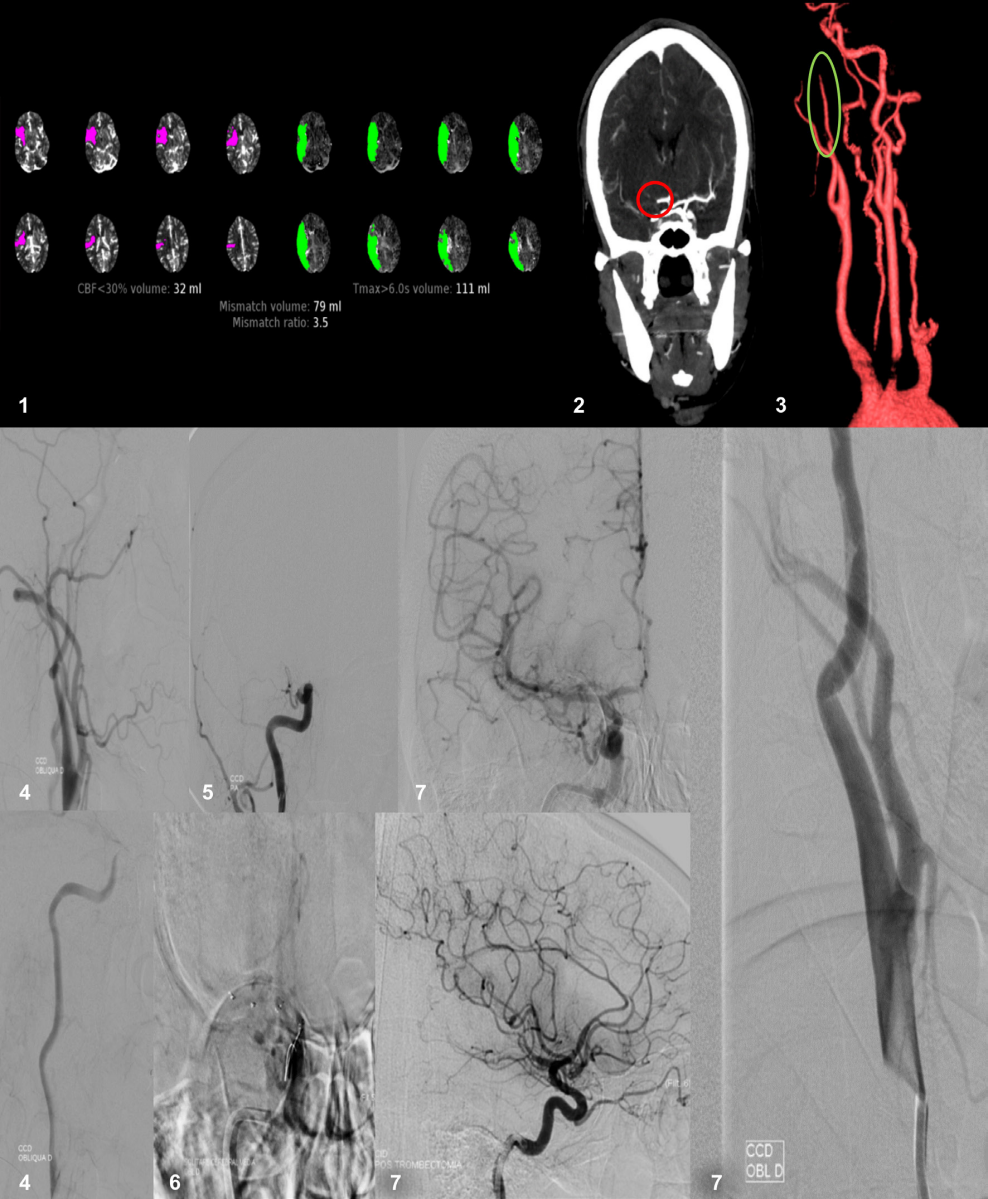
Patient that was admitted with acute stroke due to tandem occlusion and underwent mechanical thrombectomy. 1. Perfusion CT showing an acute stroke with large penumbra. 2. Median cerebral artery occlusion on CTA. 3. Internal carotid dissection with “pencil sign” on 3D CTA. 4. Angiographic image of carotid dissection and retarded contrast flow. 5. Median cerebral artery occlusion on DSA. 6. Position of stent retriever. 7. Post thrombectomy intracranial and cervical carotid images.
